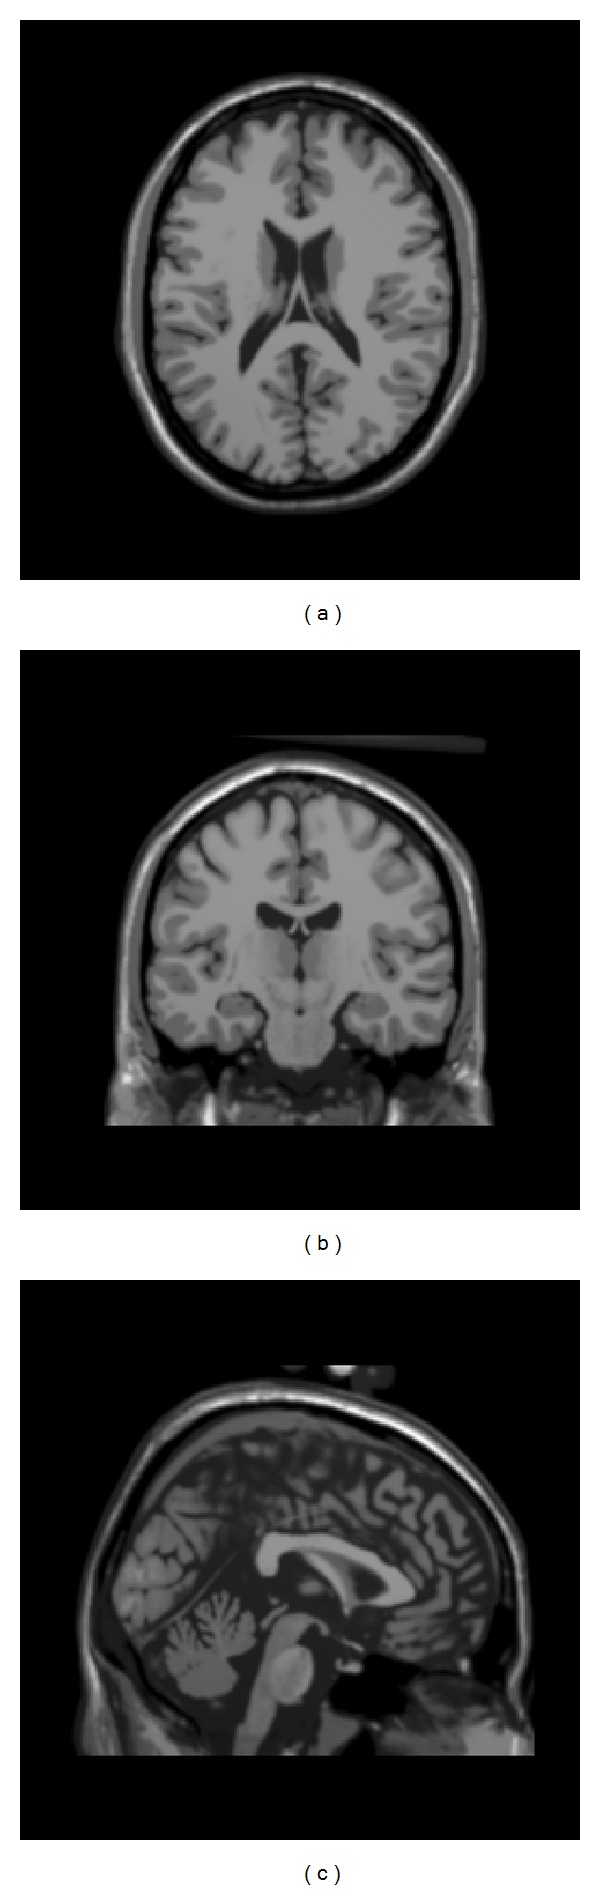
Brain MR images with three views axial, coronal, and sagittal [41].
